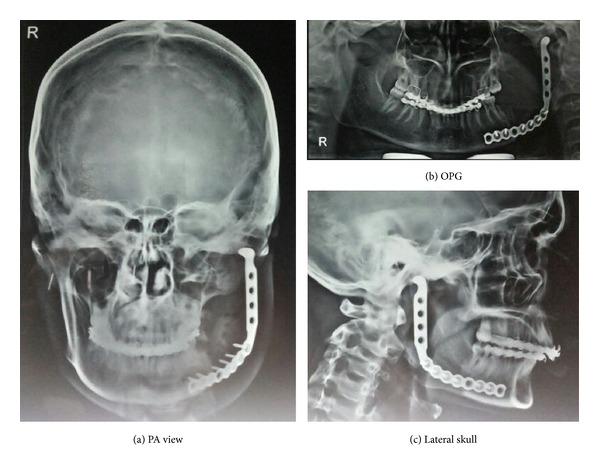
Postoperative radiographs: (a) PA view, (b) OPG, and (c) lateral skull.
